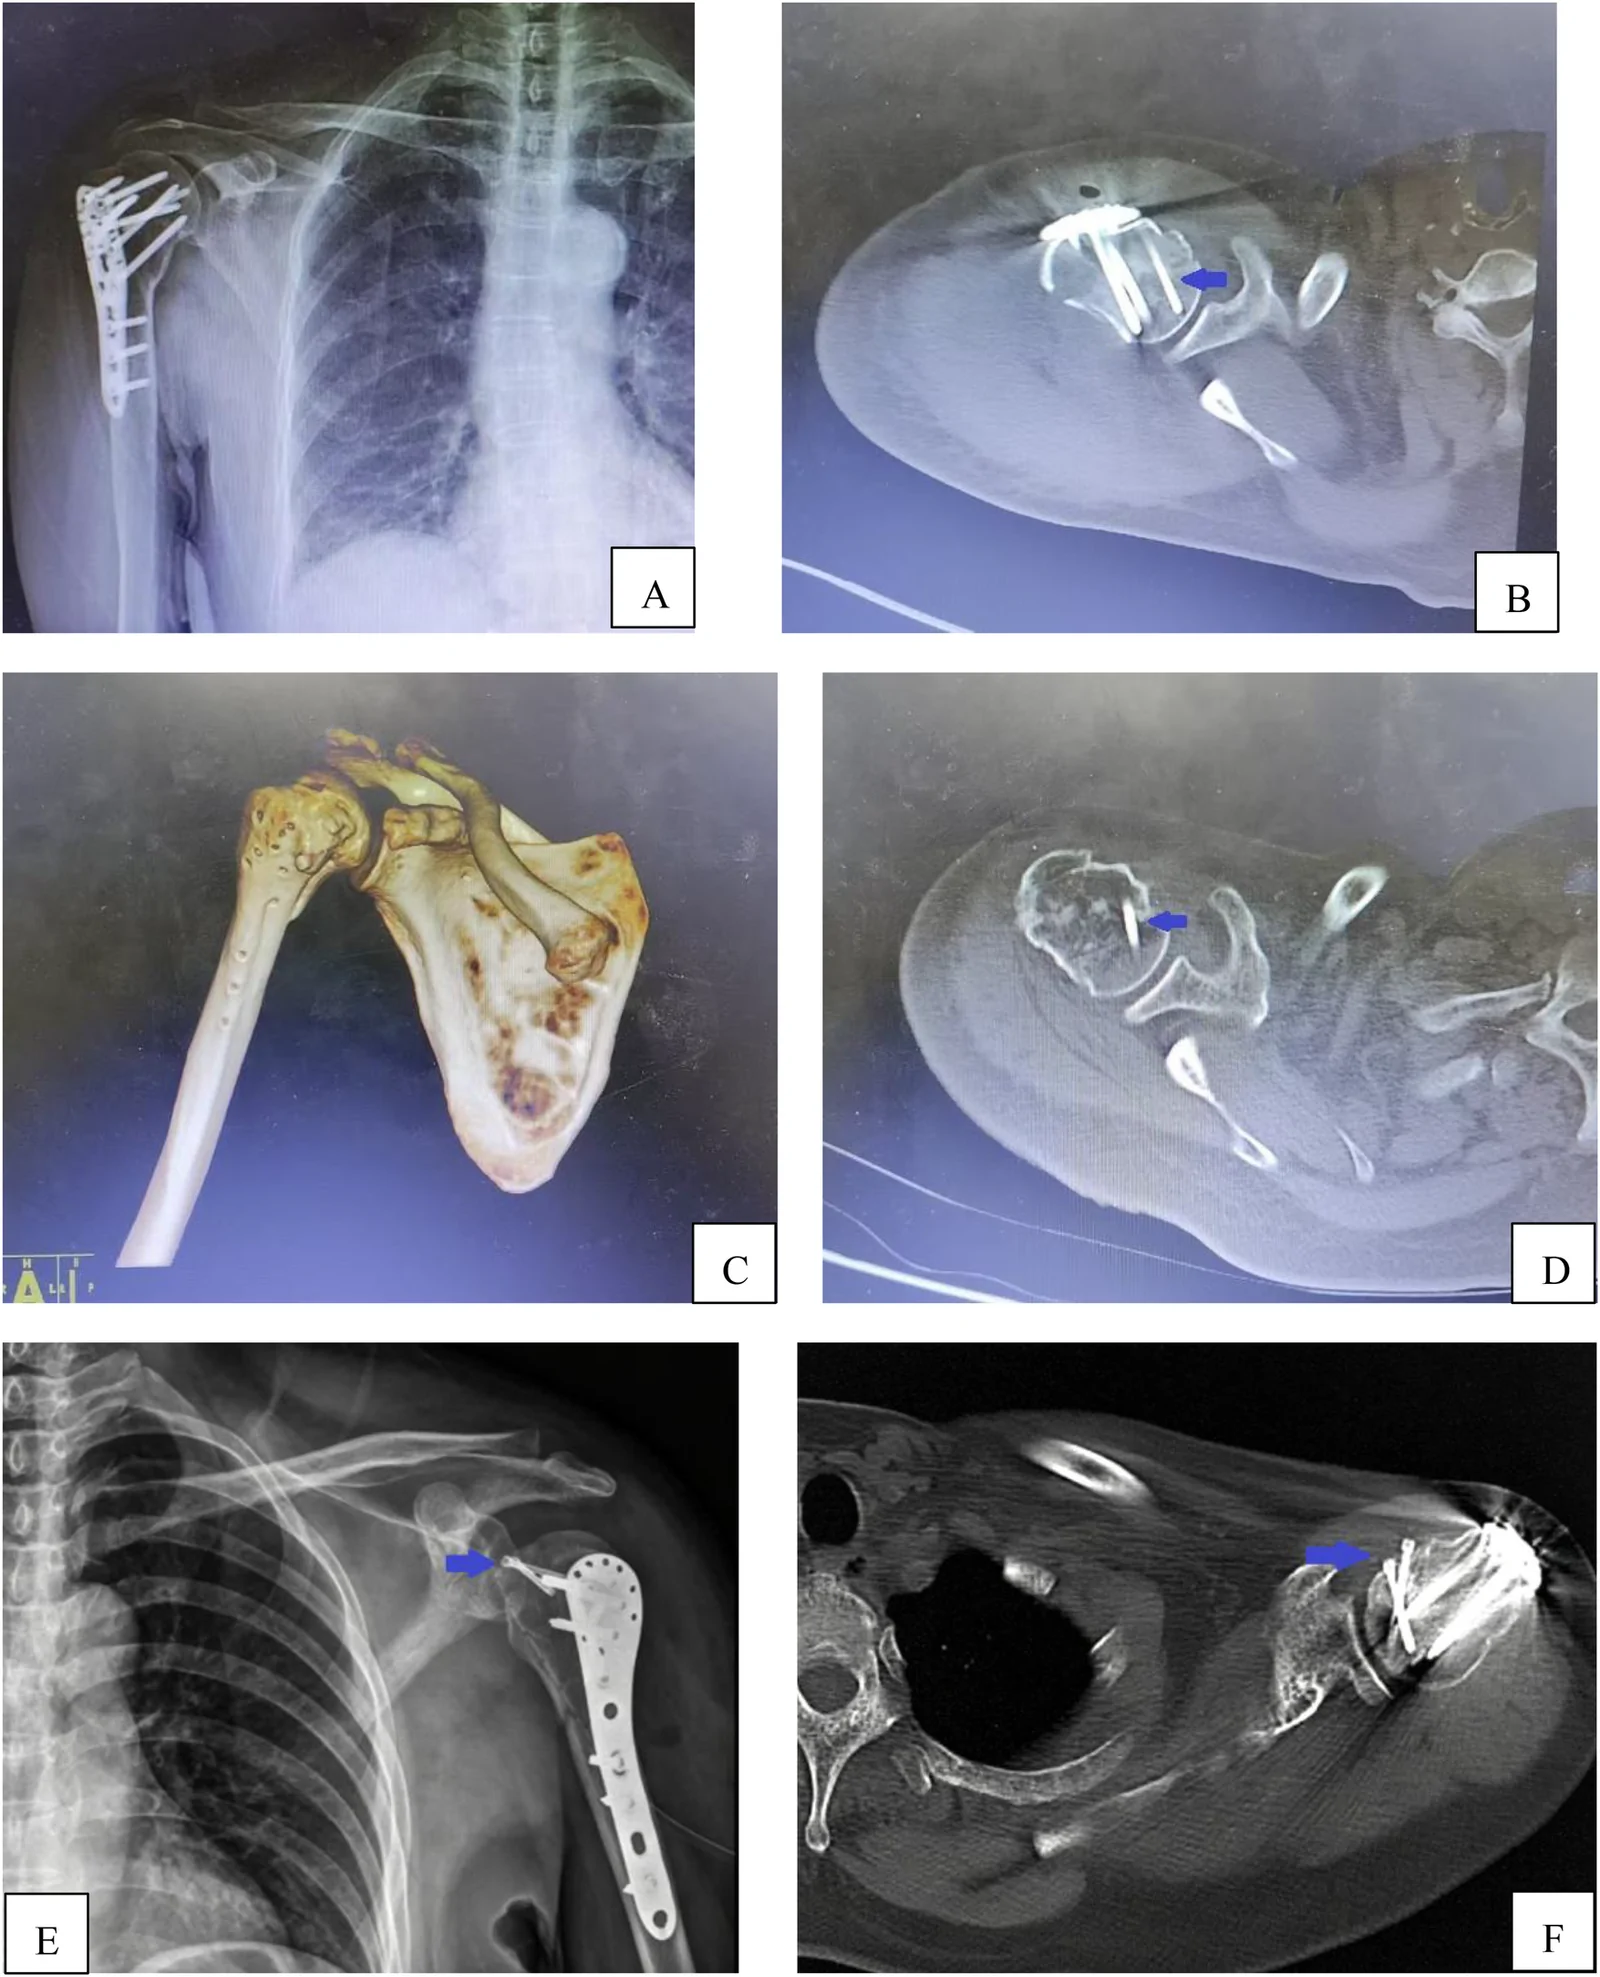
Postoperative imaging of the patient NO.4: (A) Anteroposterior x-ray. (B) Axial view of 3D CT. The blue arrow indicates the screw used to fix the bone graft. (C) 3D CT after removal of internal fixation at 1 year postoperatively. (D) The plate was removed, but the screw fixing the bone graft (blue arrow) was retained. Postoperative imaging of the patient NO.7: (E) Anteroposterior x-ray. (F) Axial view of 3D CT.
